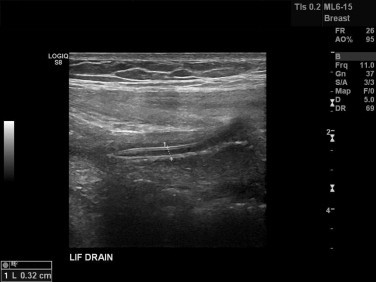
Ultrasound image showing resolution of the collection (1) around the laparoscopically inserted hernia repair mesh.
